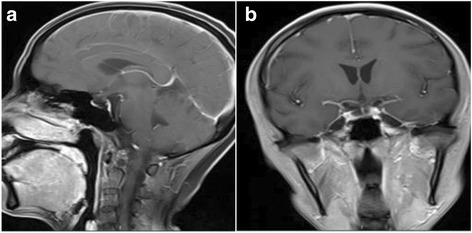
Abnormal enhancement shadow was observed in the tentorium cerebelli and basicranial sella region in a and b MRI images
